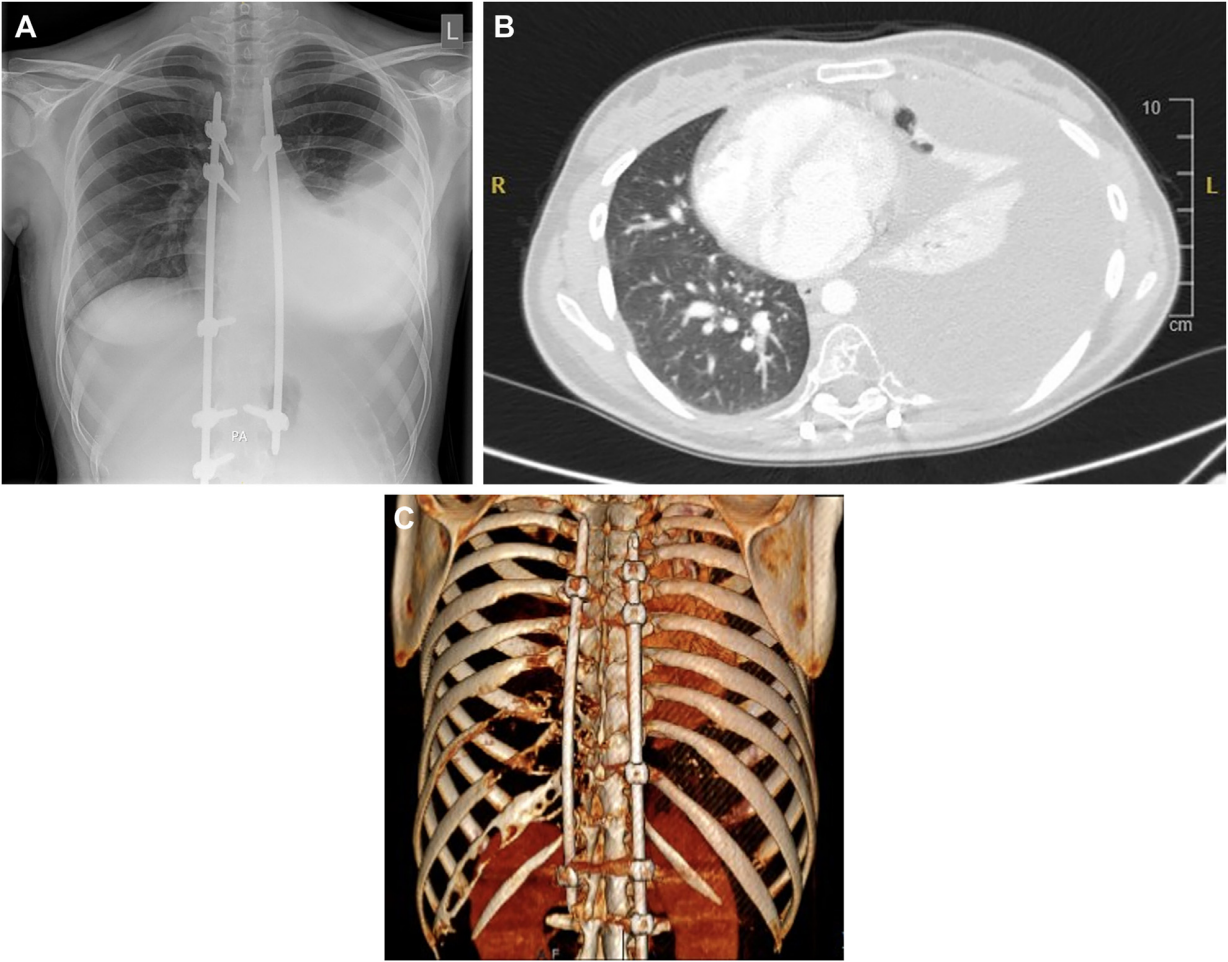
(A) Posteroanterior chest radiograph showing large left-sided pleural effusion as well as spinal rods and screws from previous surgery. (B) Computed tomography of the thorax demonstrating mediastinal shift due to the large left-sided pleural effusion. (C) Three-dimensional reconstruction showing lytic lesions dispersed throughout T7-T12 vertebrae and left ribs 8-11.
